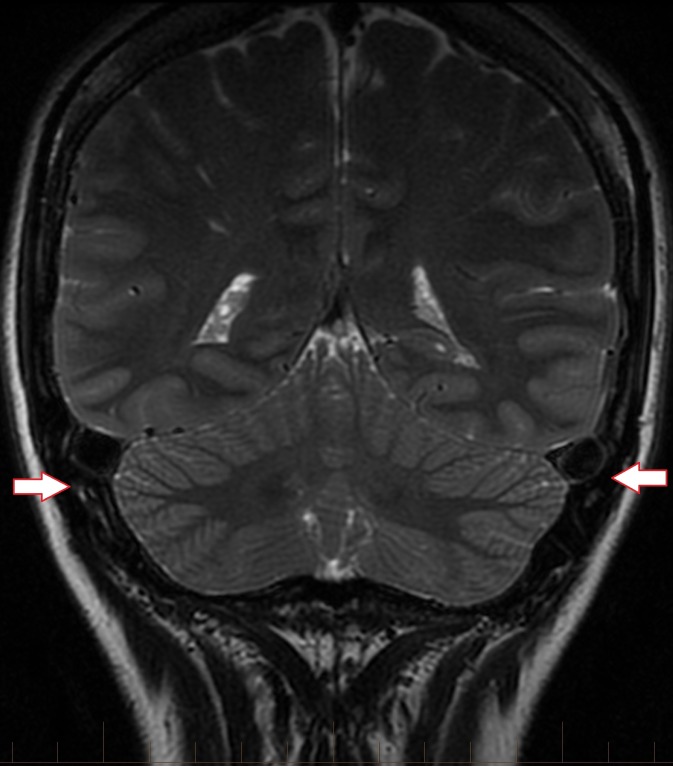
Coronal T2 brain MRI revealing an engorged appearance of the bilateral transverse dural venous sinuses
